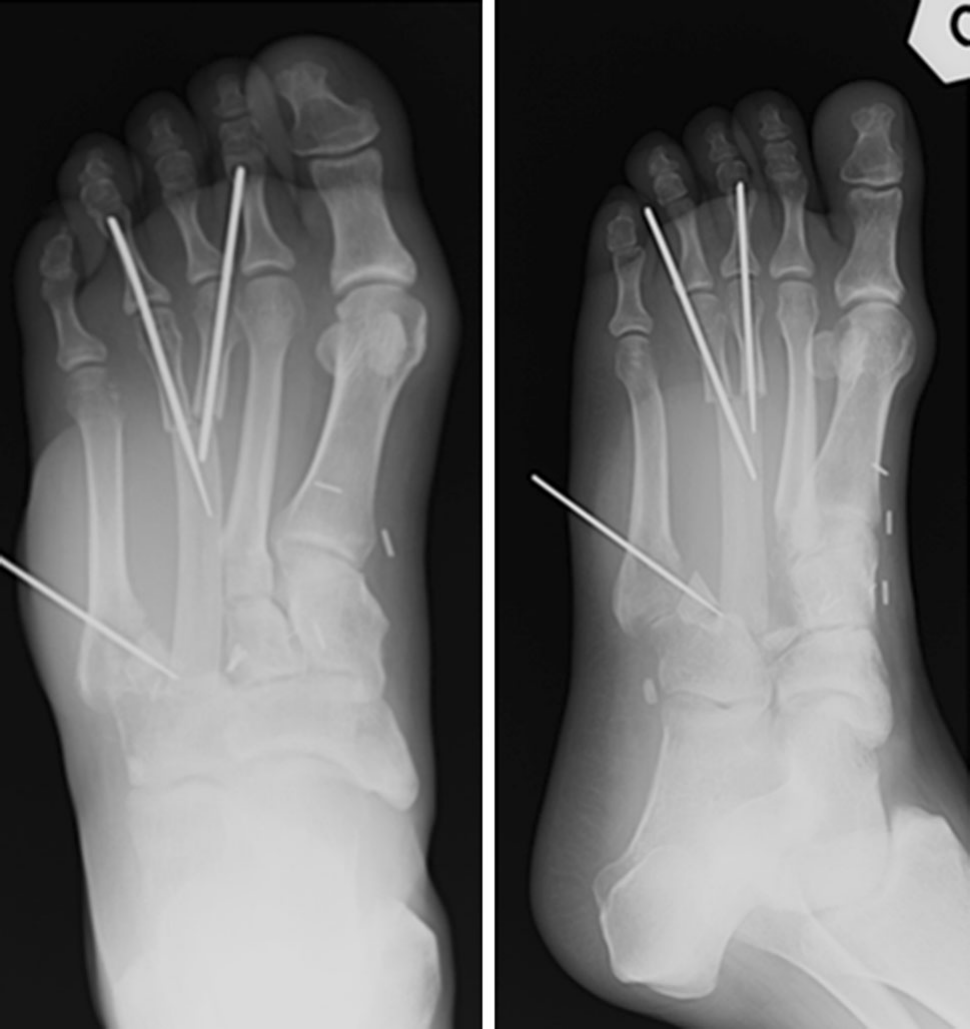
Postoperative radiographic oblique and antero-posterior view
